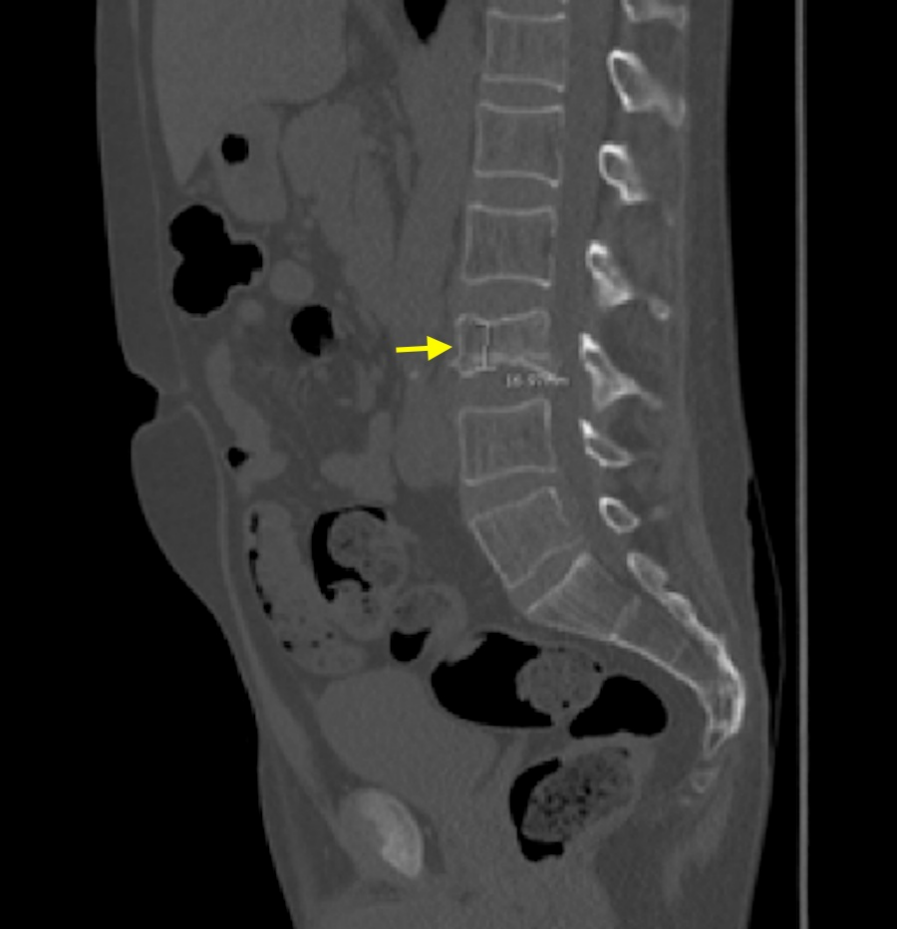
Image showing the computed tomography of generalized osteoporosis and compression fracture of lumbar (L3) vertebral body (yellow arrow)
